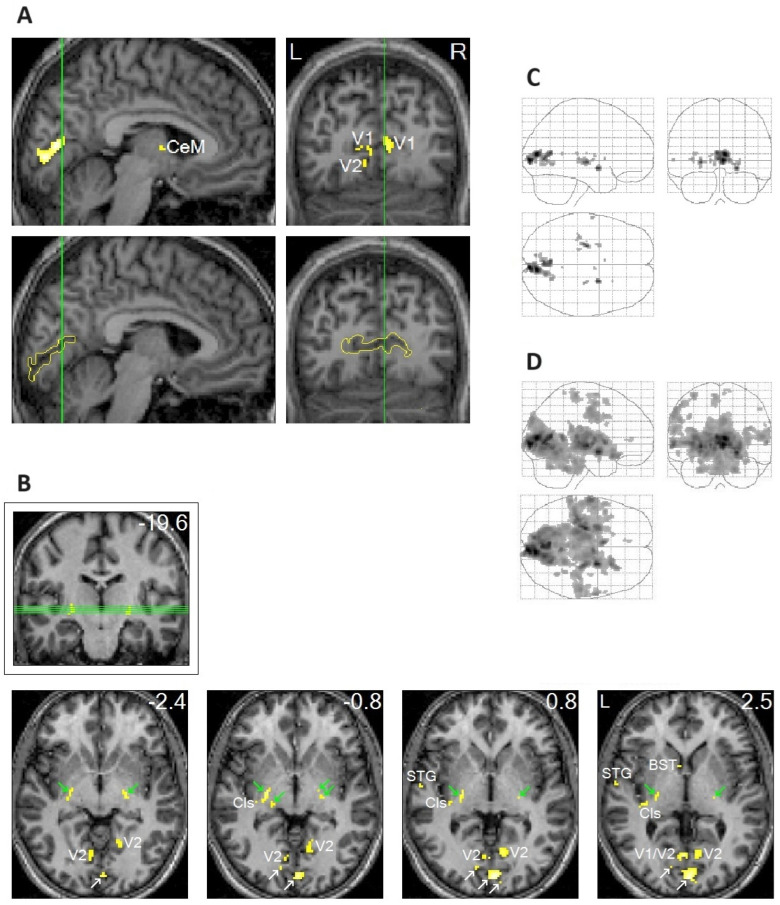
Peak rapid eye movement (REM)-locked activation in adults (functional magnetic resonance imaging study; *n* = 24, one-sample *t*-test).
